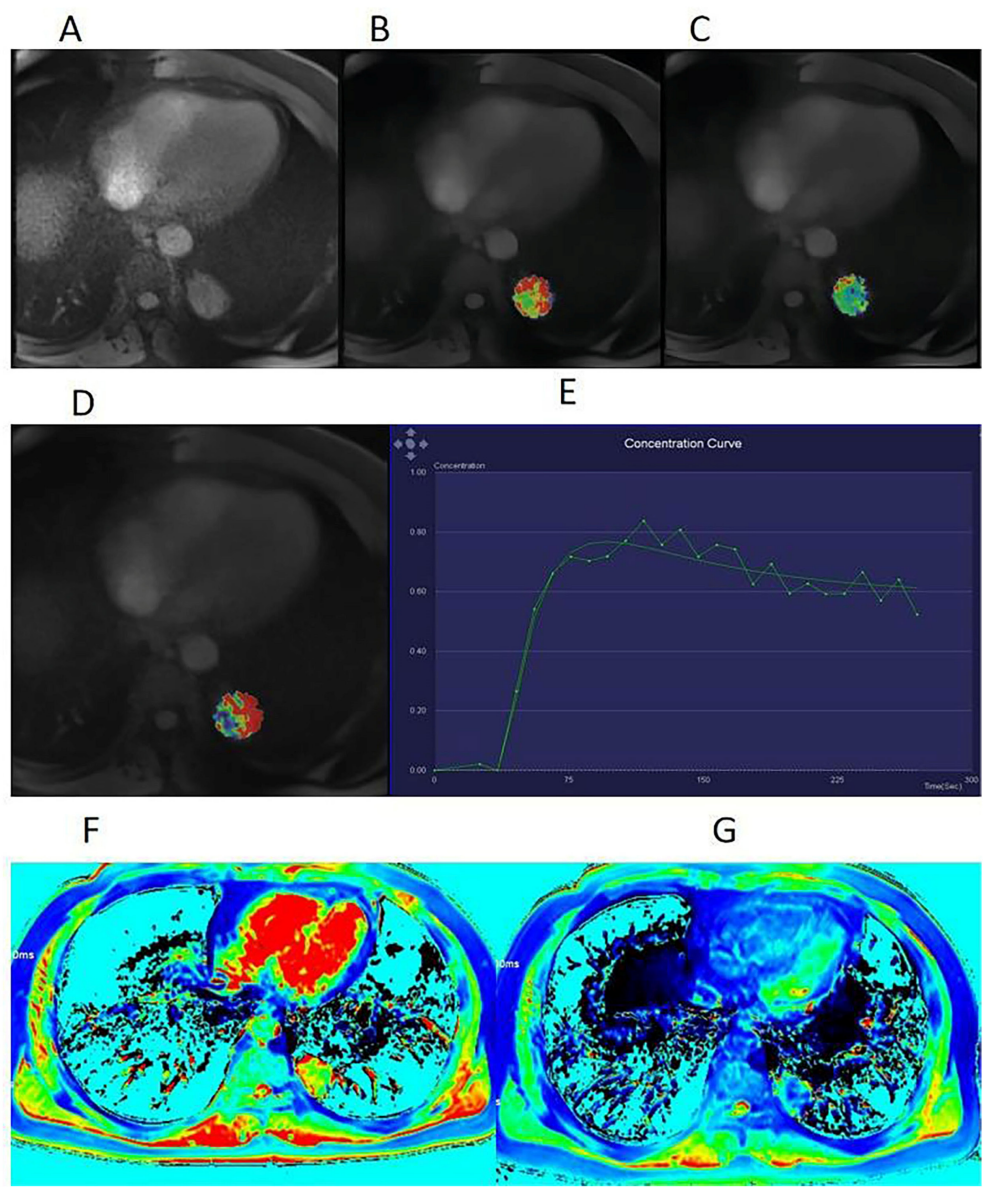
A 53-year-old man with squamous cell carcinoma (SCC). (A) Dynamic contrast-enhanced magnetic resonance imaging (DCE-MRI) scan before contrast injection, showing a lesion with a diameter of 33 mm in the upper lobe of the left lung. Volume transfer coefficient (Ktrans) map (B), rate constant (Kep) map (C), extracellular extravascular volume fraction (Ve) map (D), and mean DCE time course curve (E) obtained from the quantification of DCE-MRI scans. The tumor extracellular volume (ECV) fraction was 0.32, as calculated using region-of-interest measurement within the tumor and the aorta on a pseudo-color native T1 map (F) and a pseudo-color enhanced T1 map (G).
